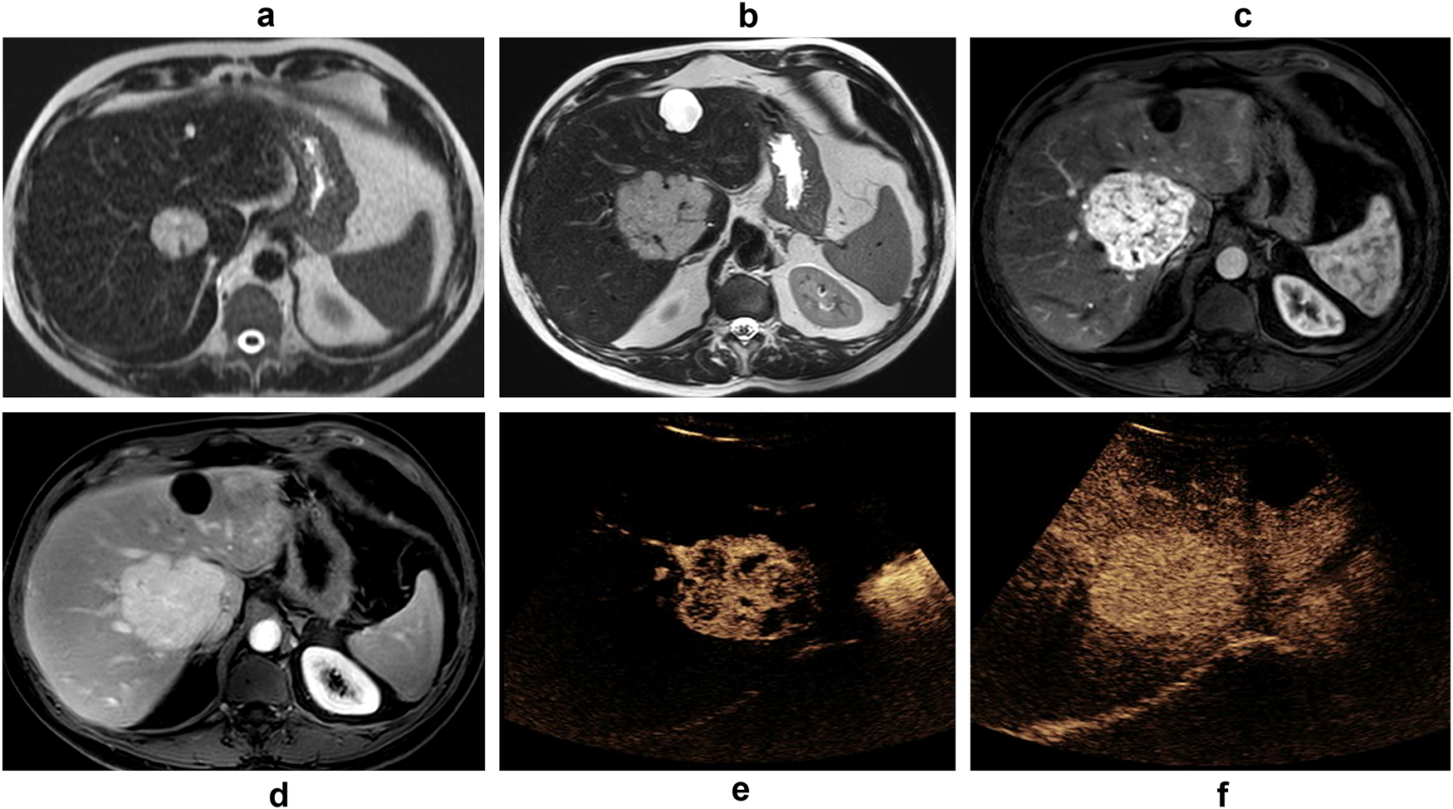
A 47-year-old male showing tumor with typical LGVNL pattern. MRI shows (a) hepatic lesion of segment VIII measuring 3.5 cm with marked hyper-intensity on fat-suppressed T2-weighted. MRI follow-up after 8 years (b–d) demonstrates a significant increase in size. The lesion measures 8 cm with marked T2 hyper-intensity (b) and serpiginous signal loss on T2 corresponding to “flow voids,” thick peripheral lobulated “flower petal shape” arterial phase enhancement (c) with progressive delayed phase enhancement (d). CEUS (e, f) shows thick peripheral lobulated arterial phase enhancement (e) with centripetal portal phase filling (f)
